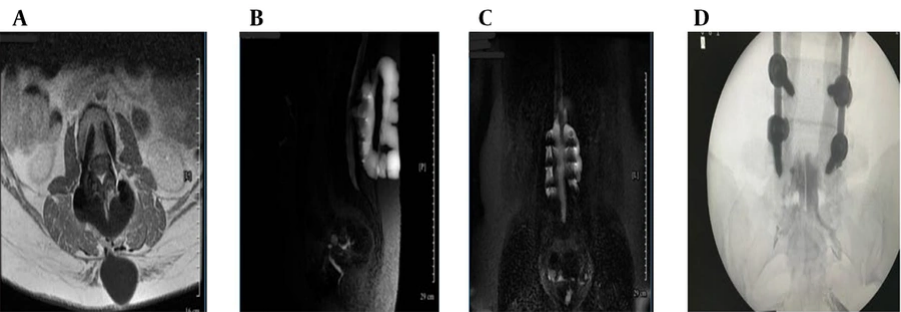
Magnetic resonance (MR) myelography and intervention (A - D): A, axial T1-weighted post-contrast magnetic resonance imaging (MRI) confirming enhancing epidural fluid; B, sagittal MR myelography showing contrast extravasation at L4-L5; C, coronal MR myelography confirming cerebrospinal fluid (CSF) tracking to sacral levels; D, fluoroscopic image showing catheter placement and cranial contrast spread.
